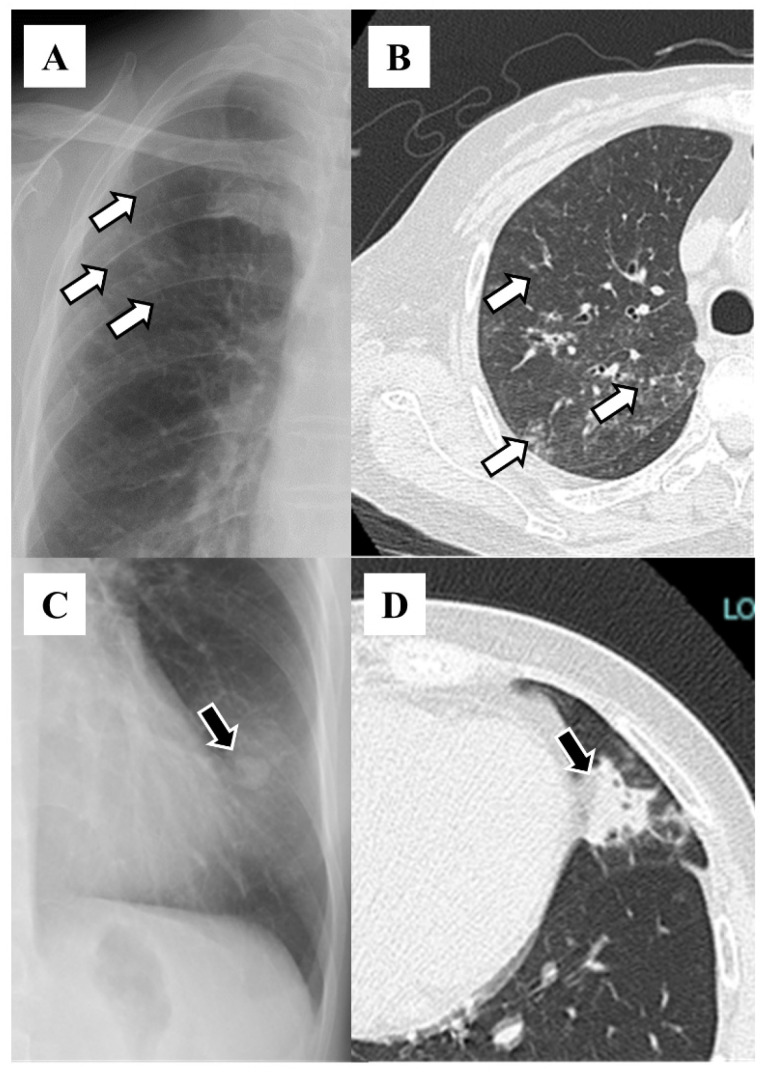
Granular shadow on chest X-ray (A) and high-resolution computed tomography (B). White arrows indicate the same lesions within the patient. Nodular shadow on chest X-ray (C) and high-resolution computed tomography (D). Black arrows indicate the same lesions within the patient.
